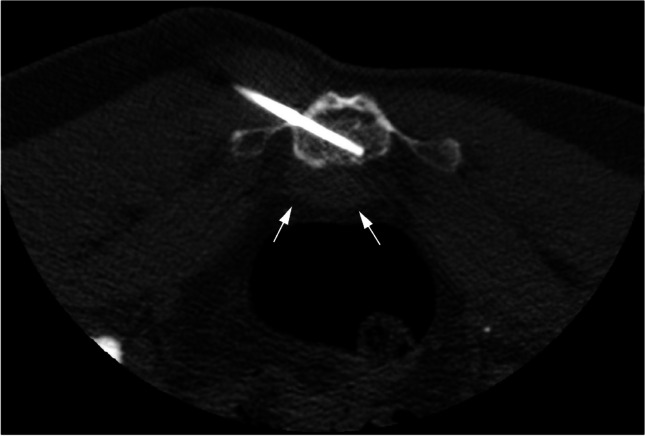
Axial image of the CT-guided biopsy (with the patient in a prone position). The biopsy was taken from the bone (see the needle tip) to prevent a false negative biopsy result from the presacral soft tissue mass which had spontaneously decreased in size (arrows)
